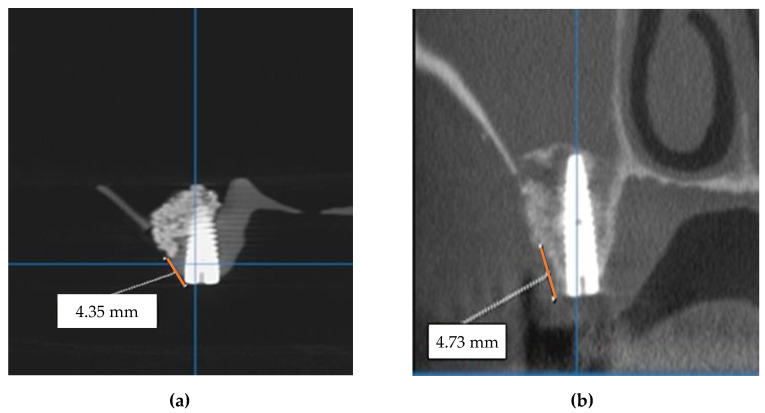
Measurement of the distance between the top of the ridge and the lowest access cut at the buccal wall of the maxillary sinus (for patient number 6 in Table 1) on (a) the training CBCT model (D2-3D) and on (b) the postoperative CBCT (D2-P) cross-section.
